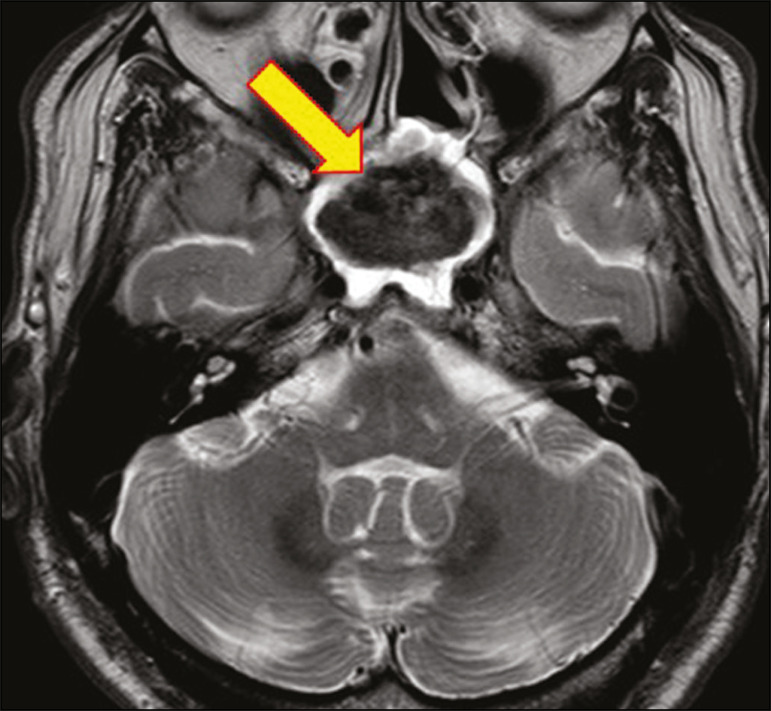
A 55-year-old patient with mucormycosis in the sphenoid sinus. Axial T2-weighted MRI sequence showing central hypointensity and a hyperintense halo.
